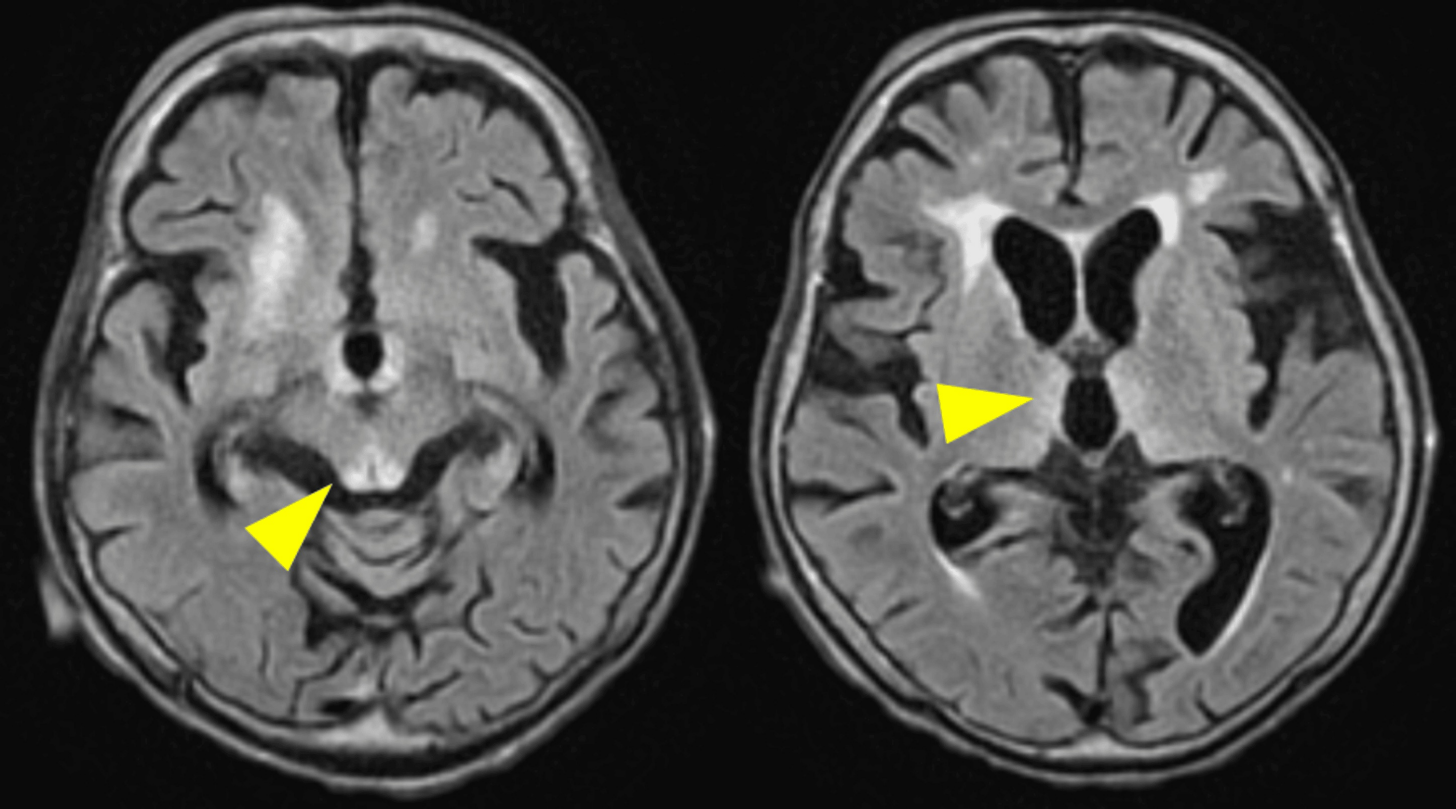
Brain magnetic resonance imaging on admission. Fluid-attenuated inversion recovery (FLAIR) images demonstrate symmetrical high-intensity lesions in the periaqueductal gray matter and bilateral medial thalami, consistent with Wernicke encephalopathy (arrows).
